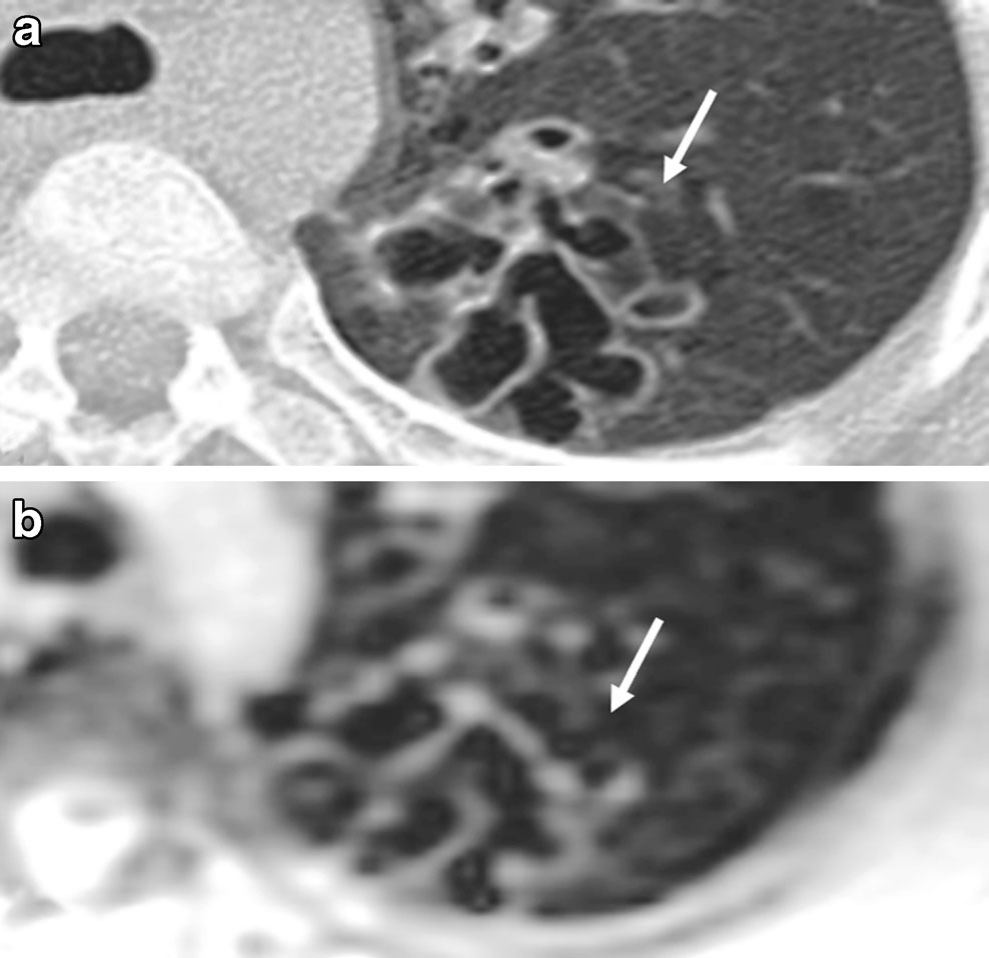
Bronchiectasis. a CT versus (b) steady-state free precession MRI in a 14-year-old boy with cystic fibrosis demonstrate bronchiectasis in the left upper lobe (*arrows*)
